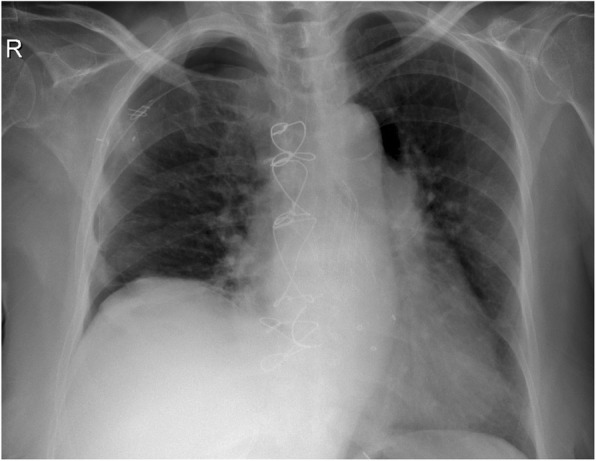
Pre-discharge chest X-ray showing the implanted aortic valve, bioprosthetic mitral valve, and tricuspid valve ring
